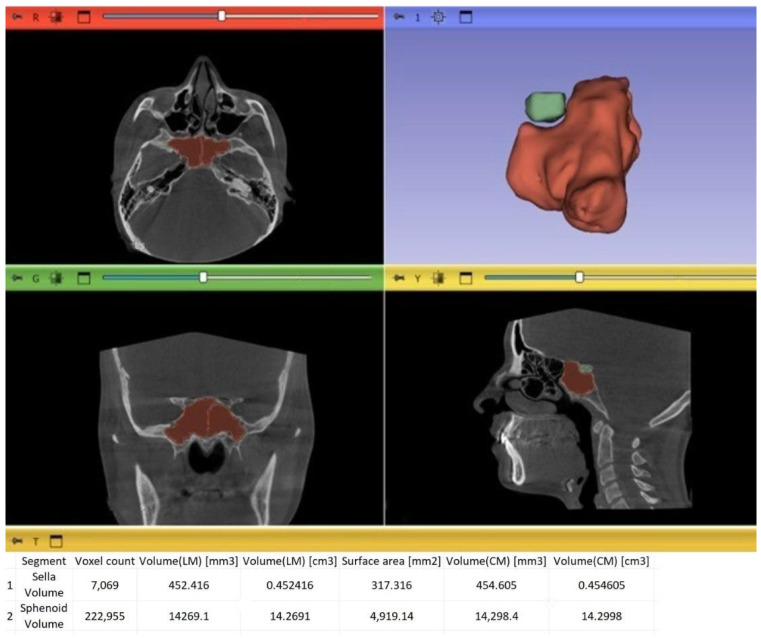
Visualization of the sphenoid sinus (red) and sella turcica (green) on sagittal and coronal CBCT slices, along with 3D segmentation.
